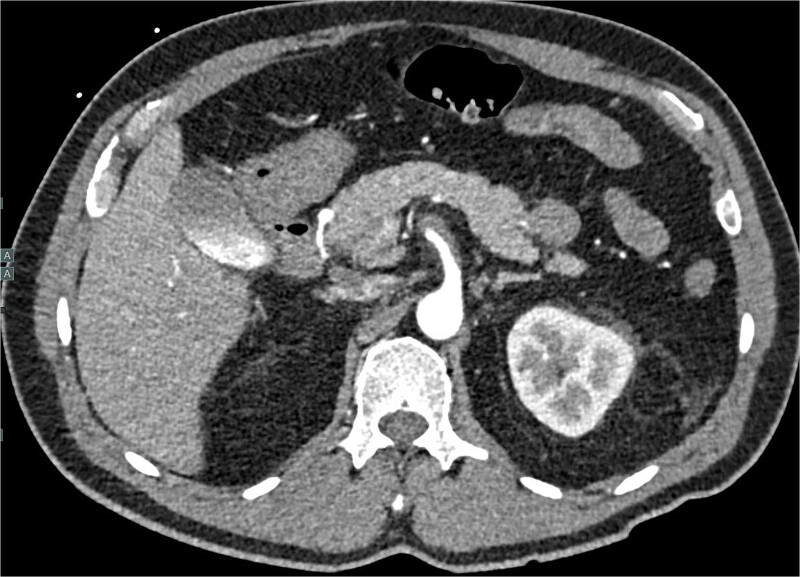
CTA abdomen and pelvis demonstrating 75% SMA stenosis secondary to SMA dissection with thrombosis of the false lumen.
